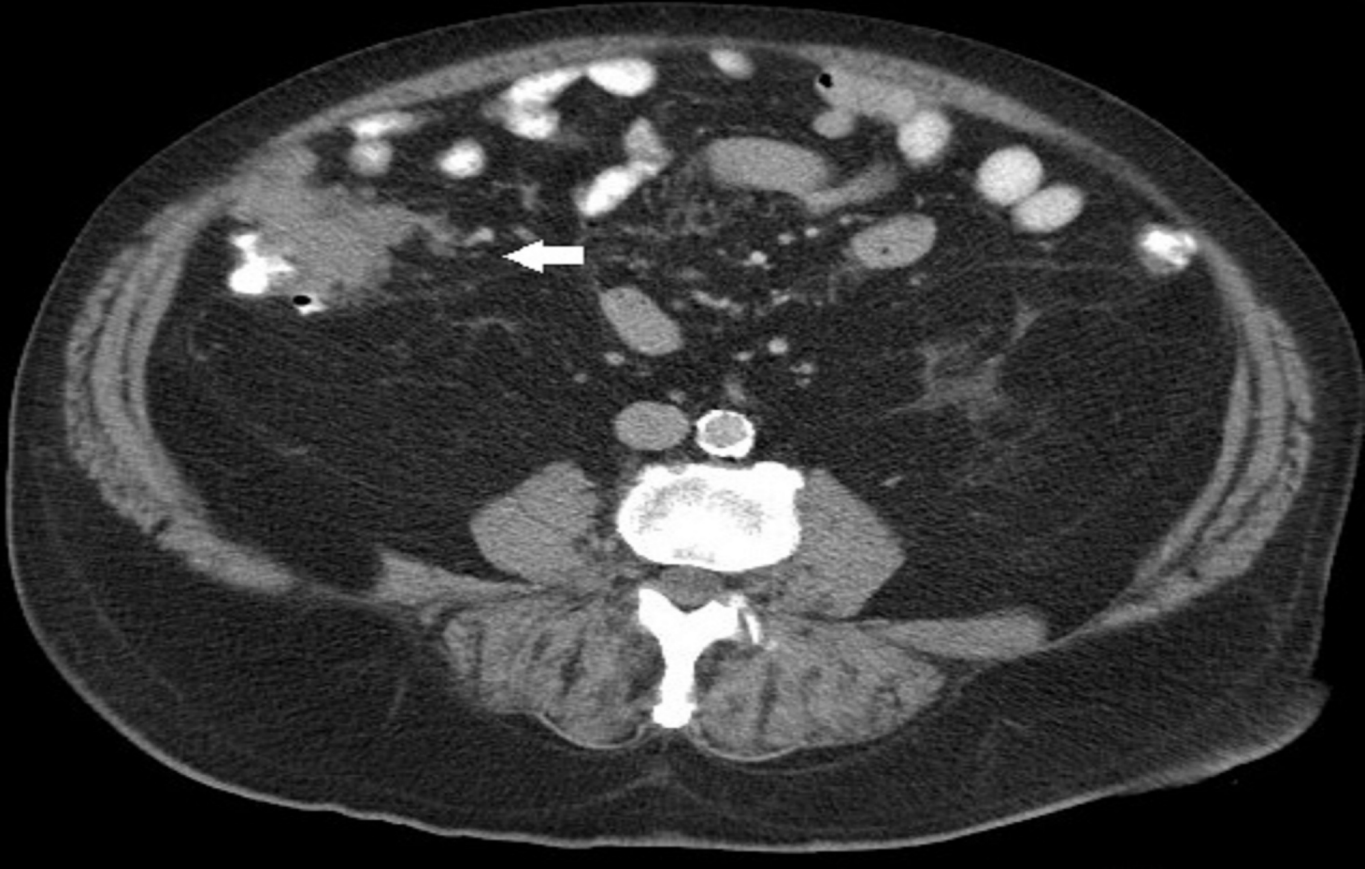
Abdominal computed tomography scan, axial view, showing an irregular 5.5 cm soft tissue mass abutting the right colon medially
